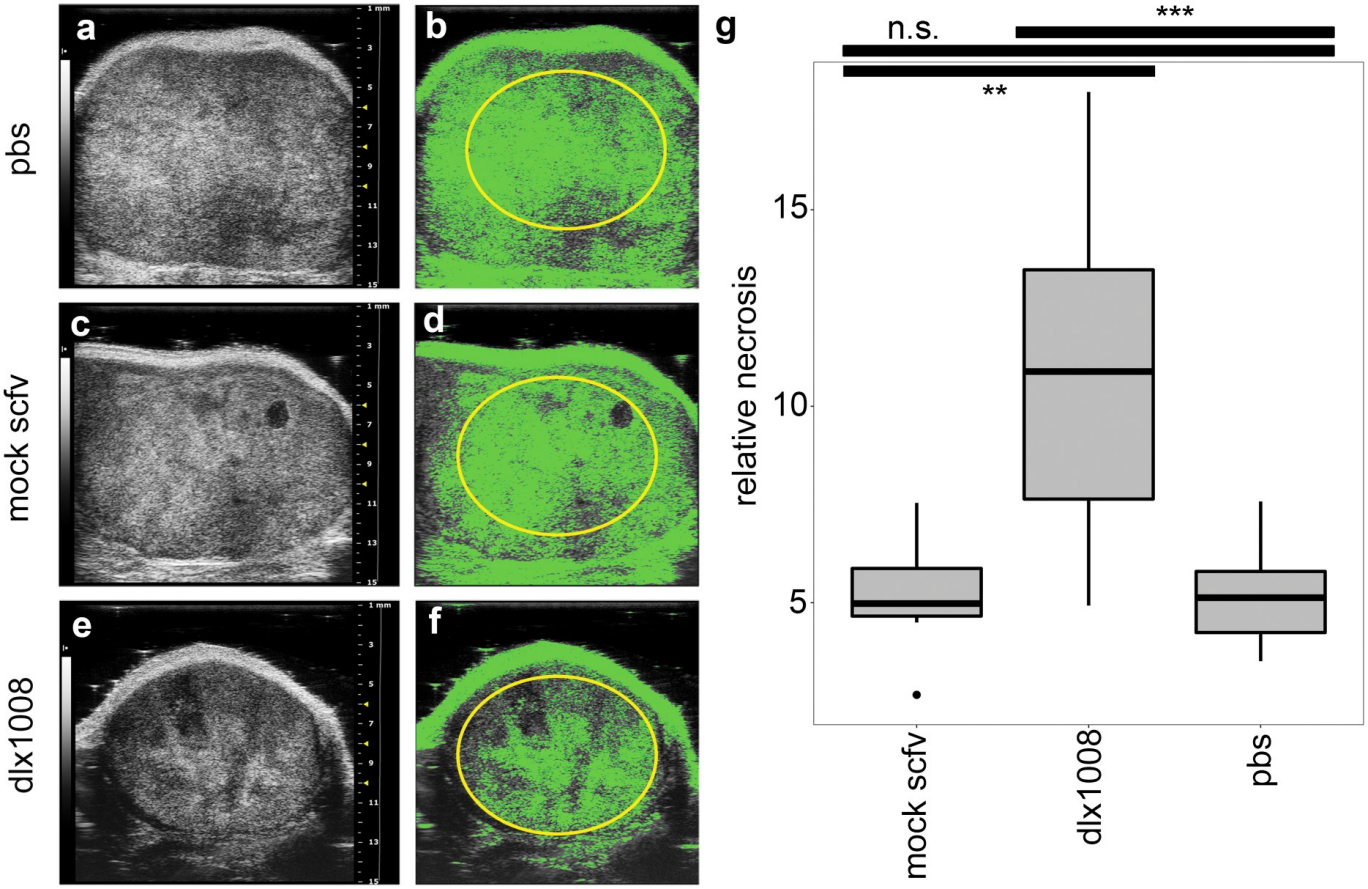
Ultrasound imaging shows decreased density in treatment tumors. B-mode ultrasound images of representative subcutaneous tumors for each group are shown in (A, C, E). Dense, non-necrotic tissue was highlighted in green using ImageJ pixel thresholding, shown in (B, D, F). Necrosis quantification was performed by excluding highlighted pixels from analysis within the selection area (yellow oval). Images are representative of mean quantification score for each group. Percent necrosis was plotted as demonstrated in (G) for all three groups, and significance was determined using multiple comparisons of means with Dunnett contrasts. DLX1008 has significantly more necrosis than mock scFv and PBS, and there was no difference between the control groups. *p ≤ 0.0001, p ≤ 0.001, n.s. = not significant.
